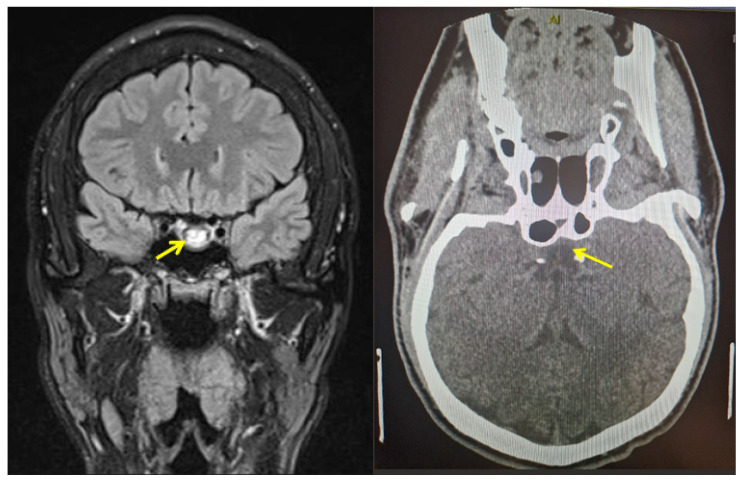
Imaging capture of a pituitary apoplexy. This is a male patient in his late 20s diagnosed with pituitary apoplexy with no prior medical or surgical history. On first presentation as an outpatient (due to severe headache), magnetic resonance imaging (performed as an emergency) shows a pituitary mass of 2.5 cm maximum diameter with inhomogeneous pattern suggesting hemorrhage (yellow arrow) at the level of a pituitary tumor (left). Intravenous contrast computed tomography (10 months since transsphenoidal surgery) shows a tendency to empty sella and no tumor remnants (yellow arrow) at the same level (right). Both captures are coronal plane.
